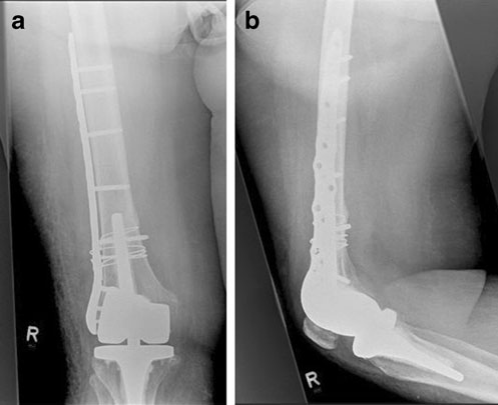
a, b Follow-up X-ray pictures at 6 months after surgery. Because of the fixation with three distal screws, one additional cerclage was set around stem and plate
